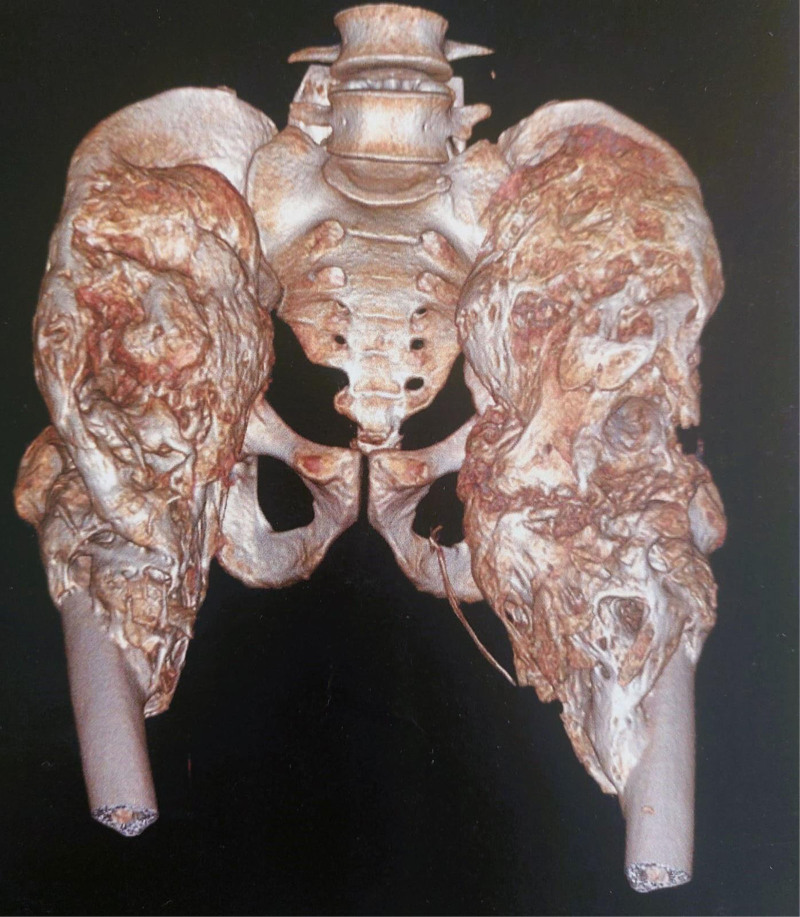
CT 3-dimensional reconstruction of the pelvis showing osteochondroma in both hips. CT = computed tomography.
